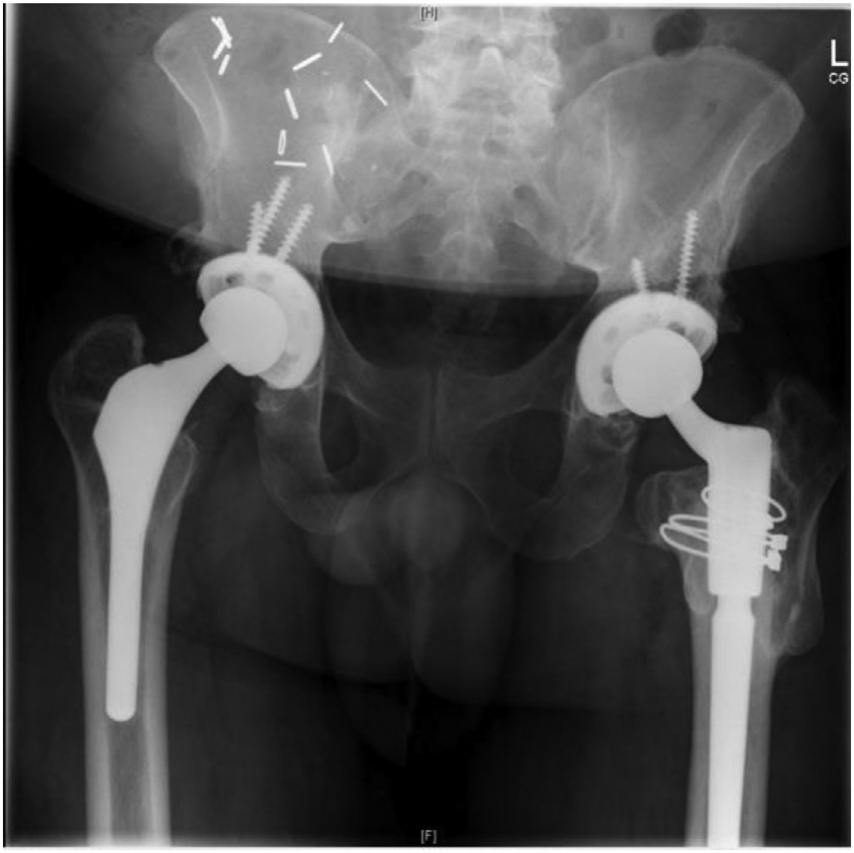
Postoperative radiographs for Patient 2-case 3, 2 years from right revision total hip arthroplasty.
